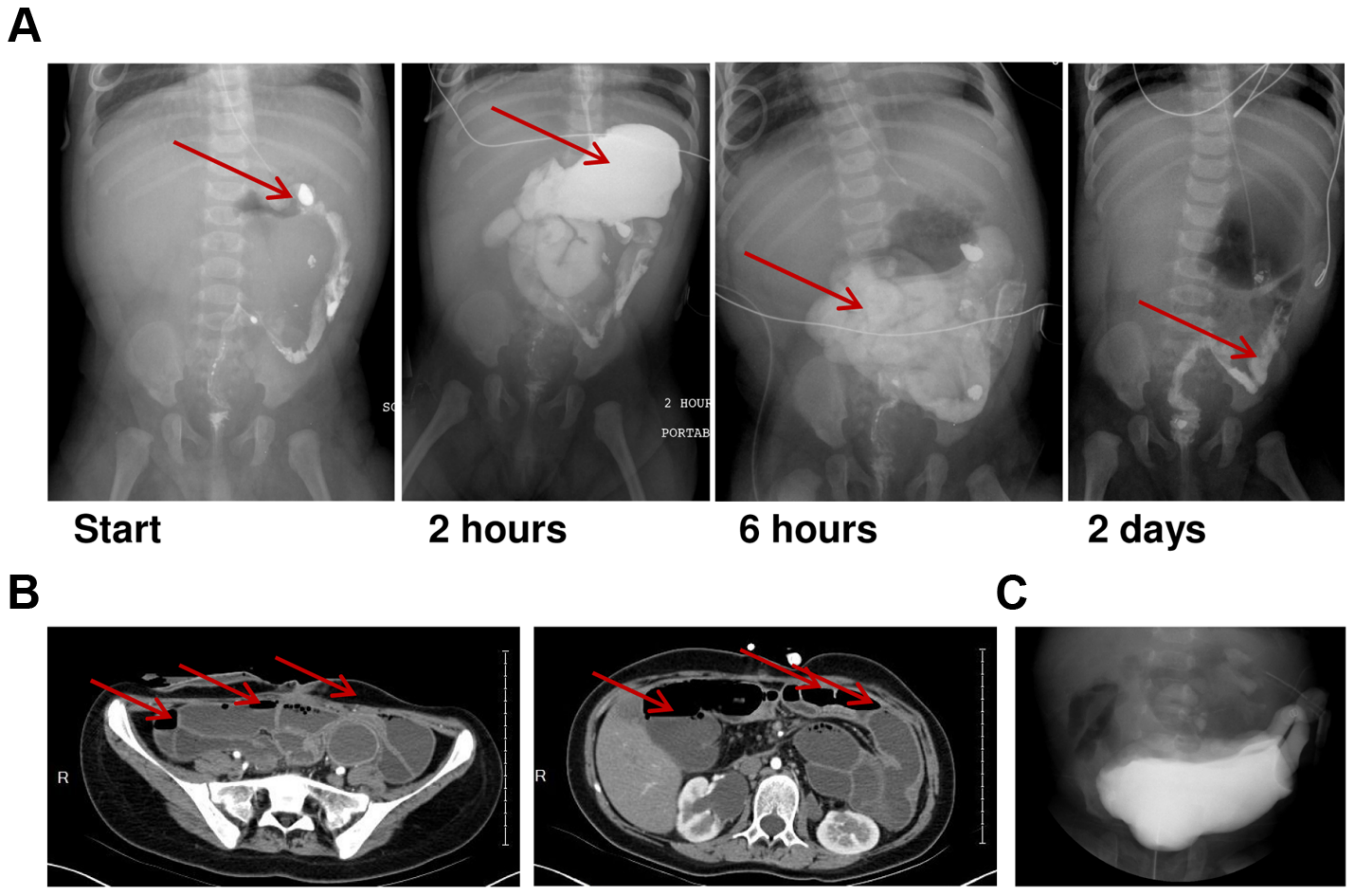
Radiologic features of MMIHS due to *de novo ACTG2* mutations. A.) An infant subject from our cohort (Fam28-1) underwent an upper GI study with small bowel follow-through. Contrast was administered beyond the pylorus (left panel, arrow) in this patient who had undergone a previous Ladd procedure. At two hours, the contrast has mostly passed retrograde back into the stomach (arrow) contrary to expectations. At six hours, the contrast is in the small intestine (arrow) and has not reached the colon. At two days, contrast material is still present in the colon (arrow) suggesting a severely delayed transit of material in the GI tract consistent with hypoperistalsis. No physical obstructive lesions are visualized. B.) An abdominal CT scan on an adolescent patient with MMIHS (Fam26-1). The patient has a diverting ileostomy, but multiple distended bowel loops with air-fluid levels (arrows) are seen at the level of the sacrum (left) and lumbar spine (right). C.) A voiding cystourethrogram on an infant male subject (Fam29-1) showing a grossly distended bladder.
